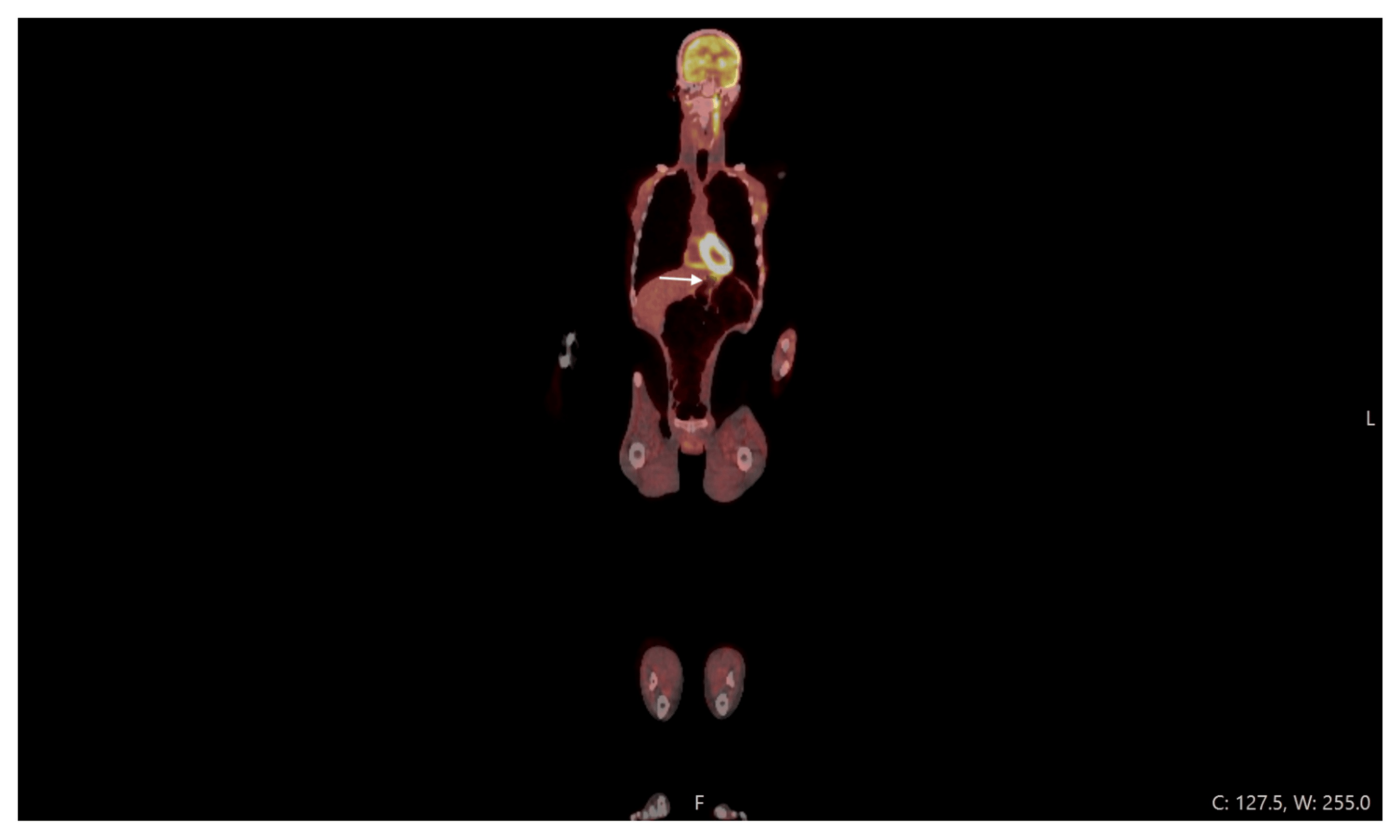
PET CT scan Diffuse gastric uptake (likely incidental finding).
